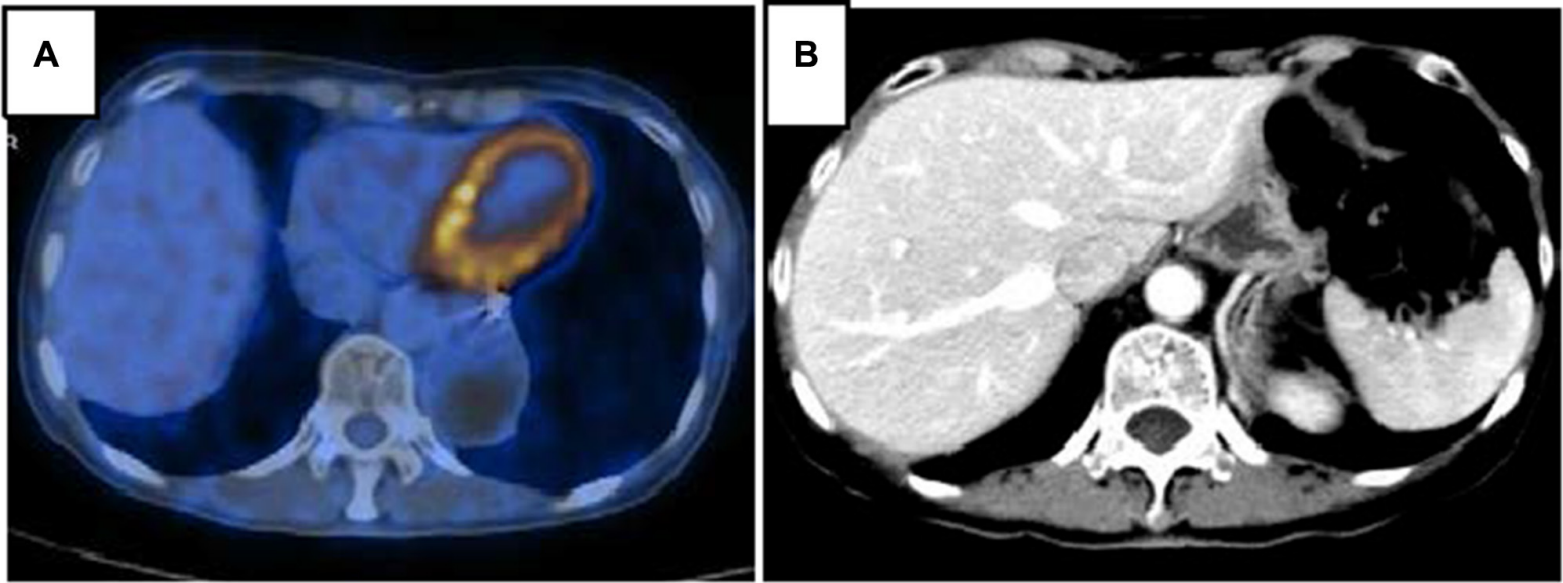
PET/CT (A) and CT with contrast (B) in follow-up care showing liver lesions disappeared after erlotinib therapy and achieved CR.
